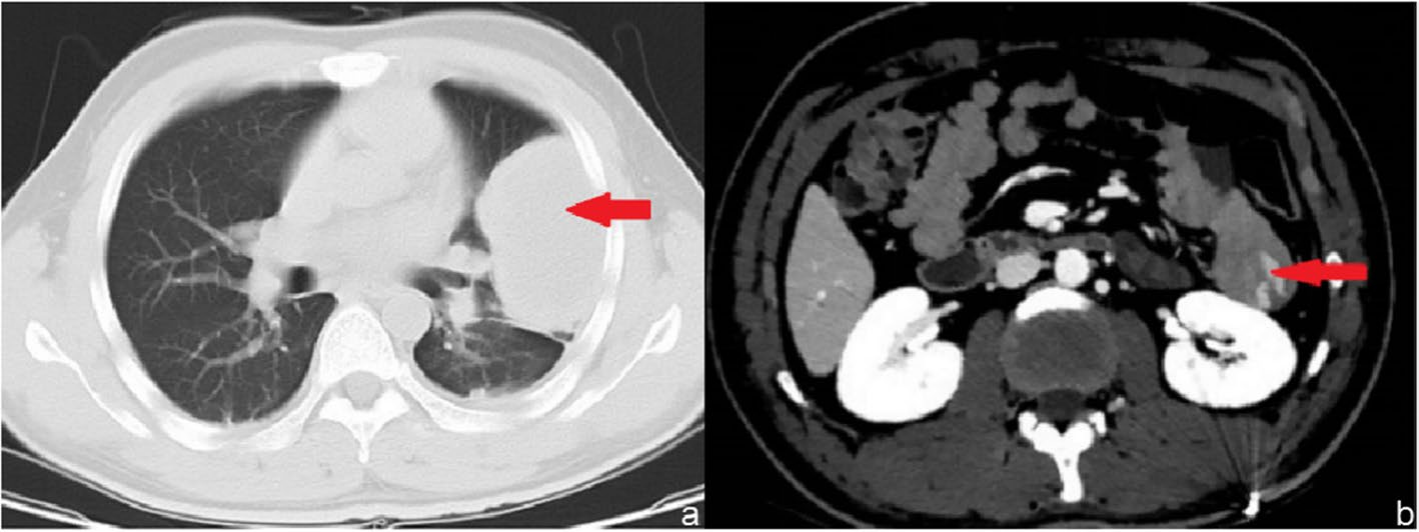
The picture a represents the chest CT results, the picture b represents the abdominal CT results, and red arrows represent the metastases
